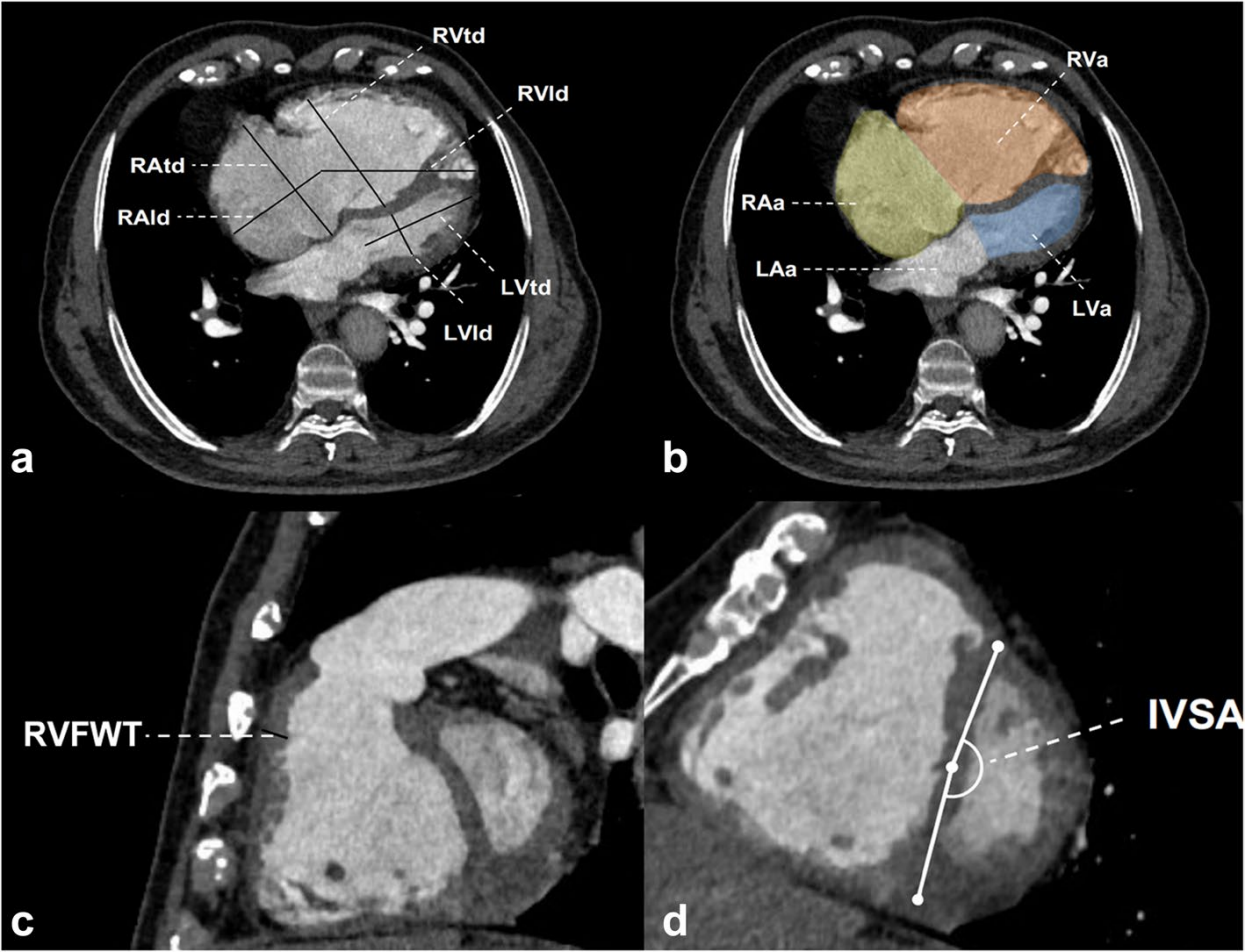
Cardiovascular metrics measured on the transversal and reconstructed views of computed tomography pulmonary angiography (CTPA). a The right and left ventricular longitudinal diameters and transversal diameters (RVld, RVtd, LVld, LVtd) and the right atrium (RAld, RAtd) and (b) the maximum four-chamber area (RVa, LVa, RAa, LAa) measured on the four-chamber cardiac view. c The right ventricular free wall thickness of the right ventricular outflow tract (RVFWT) and (d) the interventricular septal angle (IVSA) measured on the short axial cardiac view
